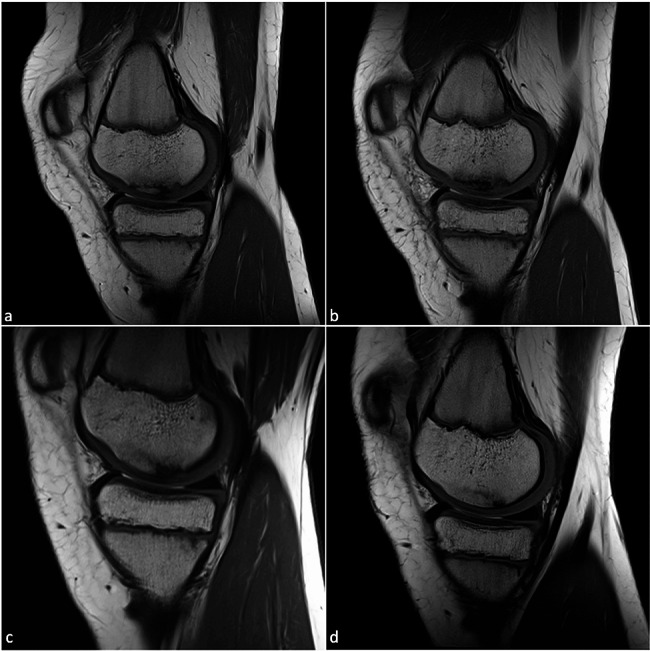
T1-weighted turbo spin echo sequences (T1-TSE) follow-up MR images in the sagittal plane of osteochondrosis dissecans of the left knee joint of twin 2 after *(a)* first clinical presentation, *(b)* three months, *(c)* seven months and *(d)* 12 months. The cartilage cover is intact at all times, the defect size is significantly reduced over time and the bone structure appears almost completely regenerated in the last MRI
